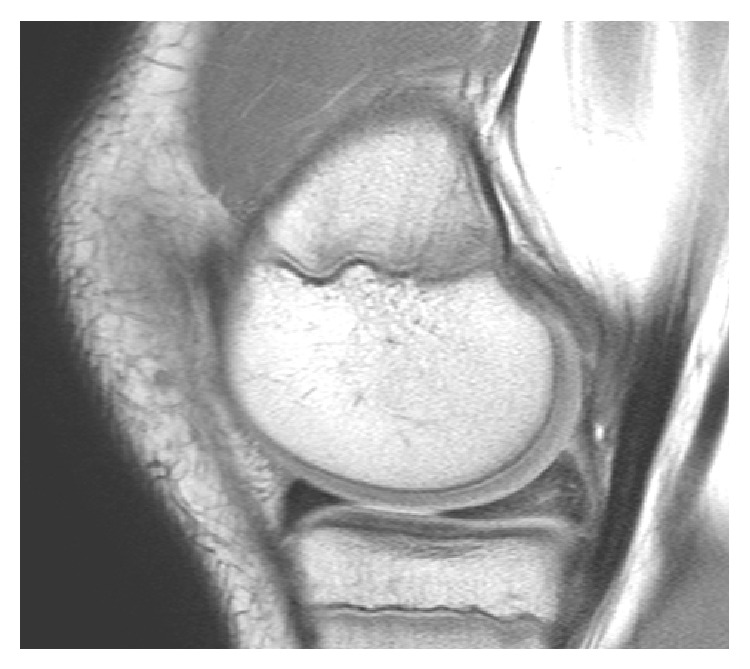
Sagittal MRI of the left medial meniscus with intrasubstance degeneration in the posterior horn.
